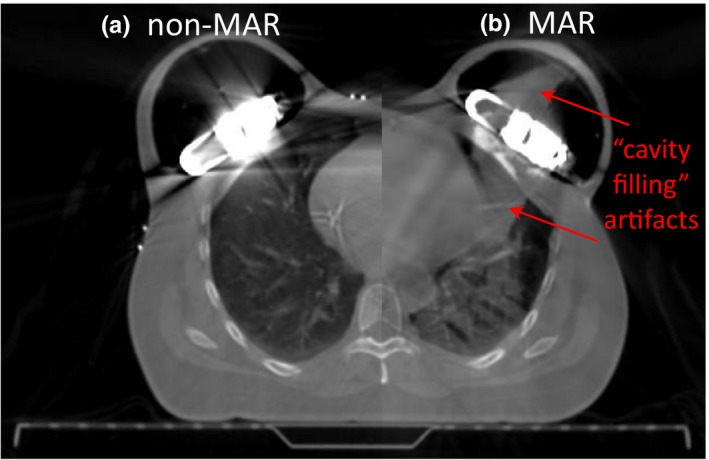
CT of a patient with bilateral AeroForm implants, split window between (a) non‐MAR and (b) MAR CT reconstructions. MAR causes “cavity filling” artifacts in the expander balloon and lungs.
